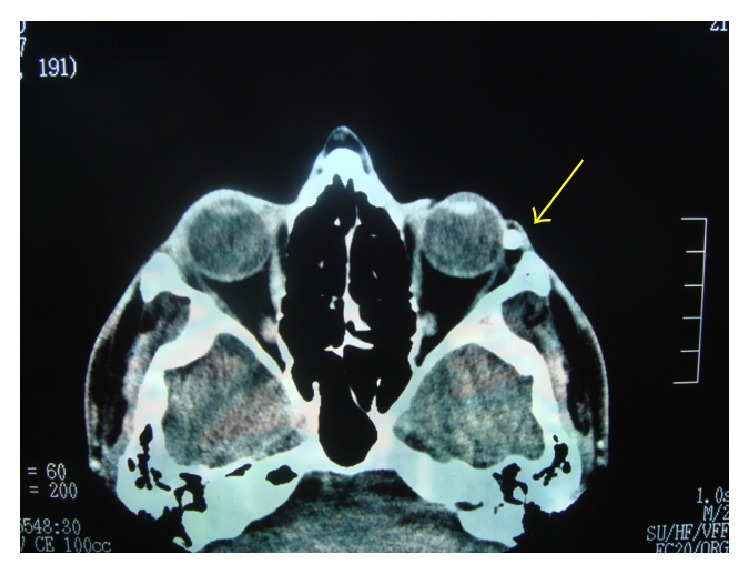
Episcleral osseous choristoma on the lateral canthus of left eye in computed tomography (arrow).
